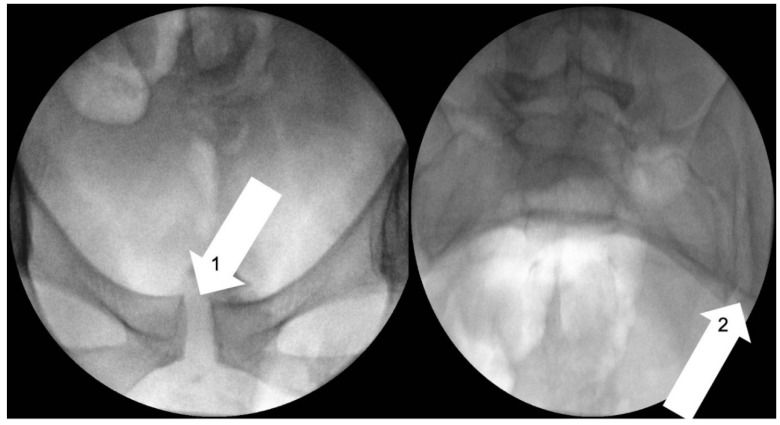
Fluoroscopic stress pelvic views radiograph in a 30-year-old female suffering from hypermobility syndrome demonstrating widening of pubic symphysis (Arrow 1) and left sacroiliac joint (Arrow 2).
